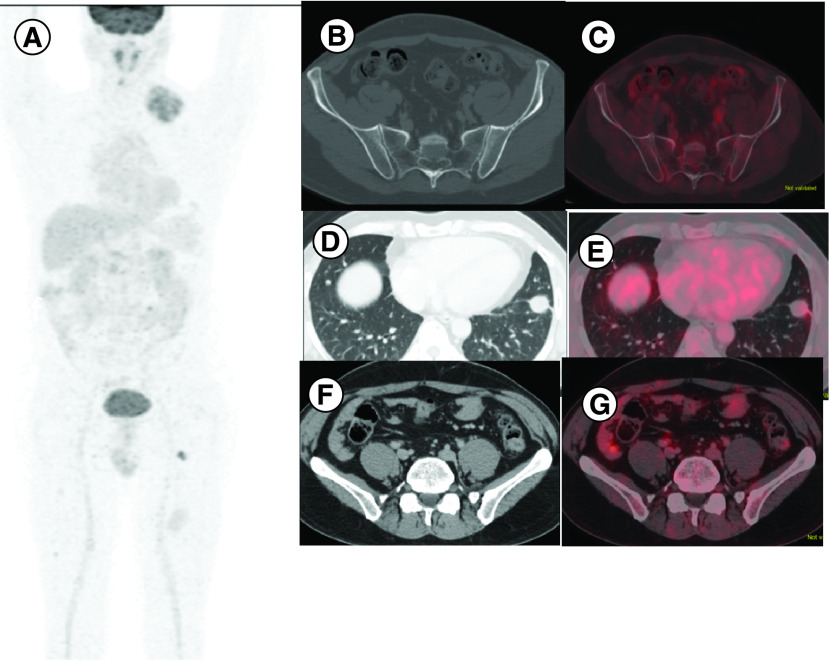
Axial contrast-enhanced computed tomography thorax of patient no. 9 showing bilateral lower lobe fibro consolidatory changes with fused positron emission tomography-computed tomography image showing mild tracer uptake suggesting drug-related changes.
